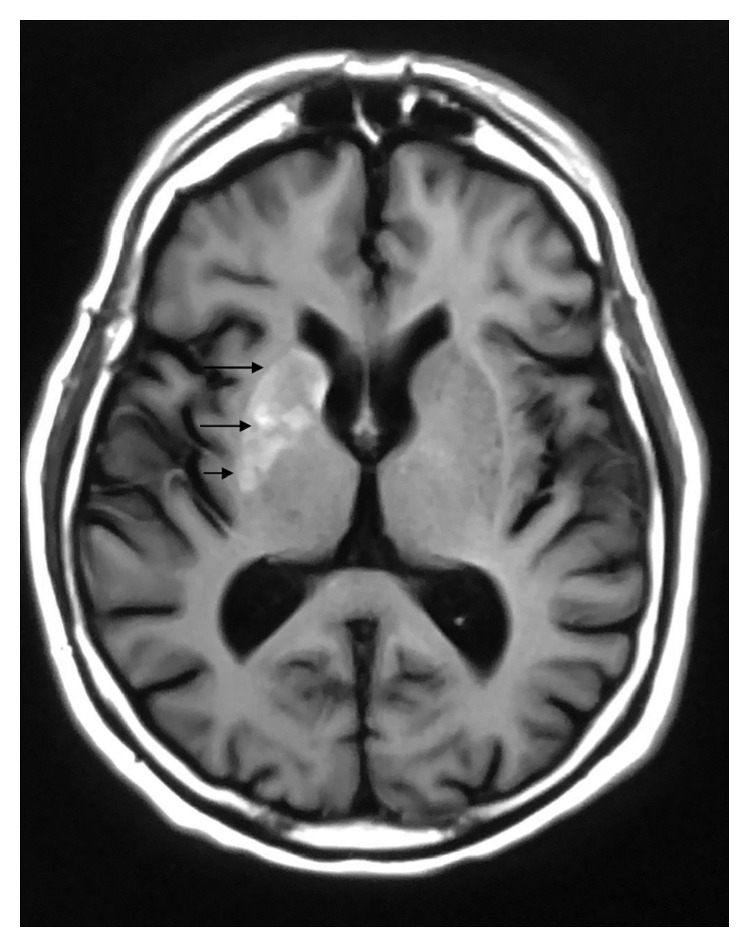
MRI brain precontrast T1-weighted axial spin echo image. Arrows highlight the right basal ganglia showing abnormal hyperintense signal.
